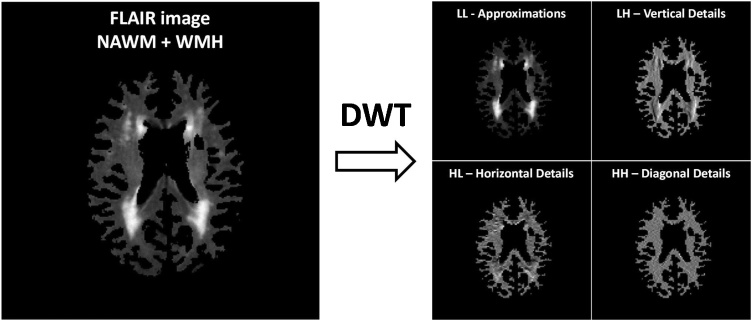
First DWT level of decomposition of a FLAIR image of the white matter tissue (NAWM and WMH) of a single brain slice.
